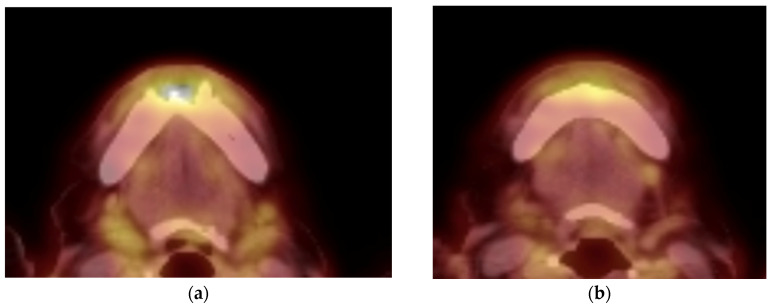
Radiologic examination of treatment effect of patient #3: (a) pretreatment and (b) post-treatment axial fused PET/CT image showing decreased avidity of the anterior mandible of SUV 5.9 to 4.4, respectively.
